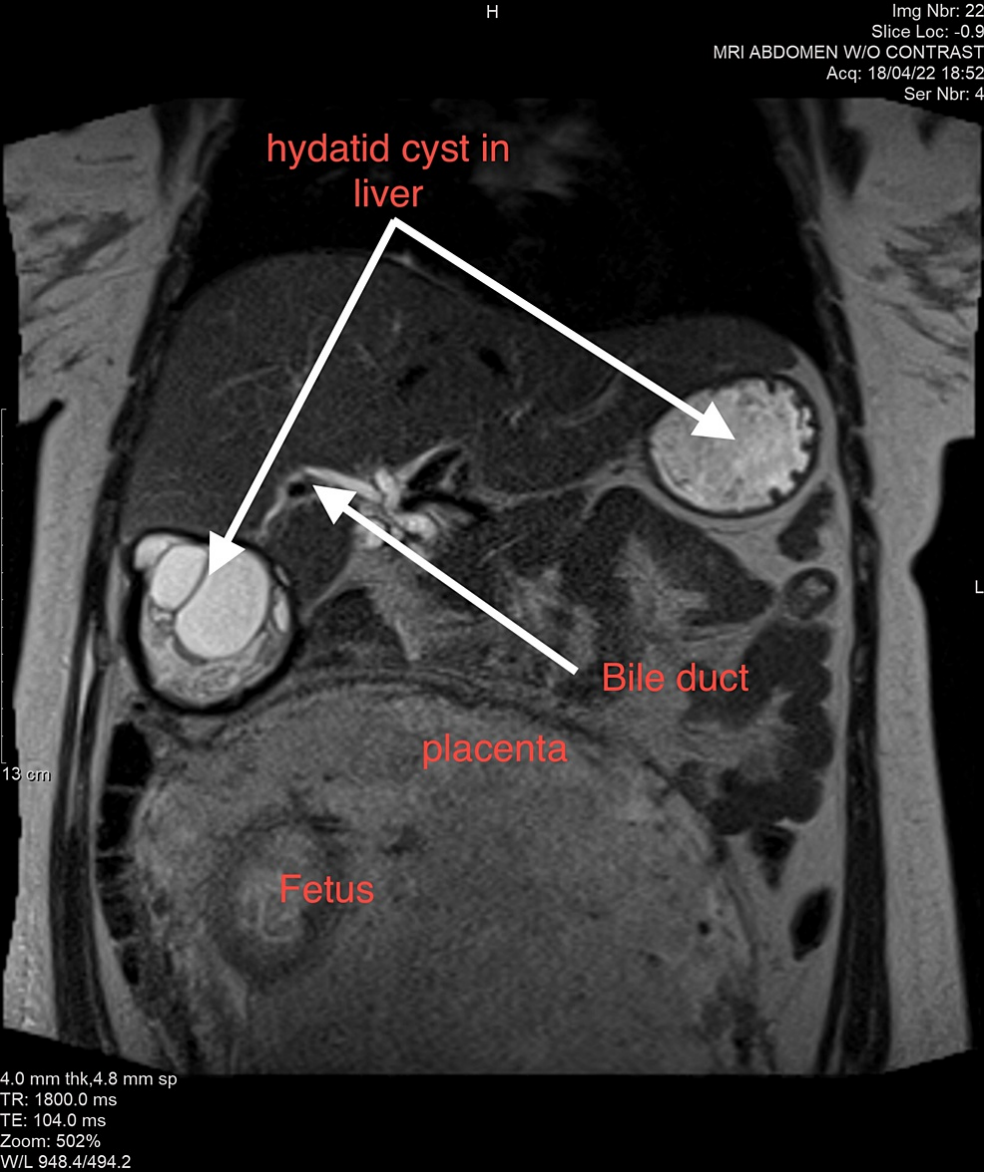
Non-contrast T2 MRI sequence of a pregnant patient, at 34 weeks and 5 days showing two liver hydatid cysts communicating with a biliary tract (double arrows). The fetus and placenta can be seen as well.
